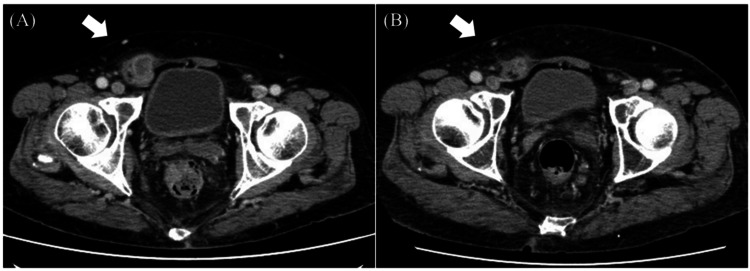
Computed tomography (CT) images after excision of the scrotal contents (A) CT scans showed wall thickening near the inguinal canal with surrounding adipose tissue inflammation (arrow). (B) Following the administration of oral ampicillin and minocycline, a reduction in inflammation was observed, as evidenced by the CT findings (arrow).
